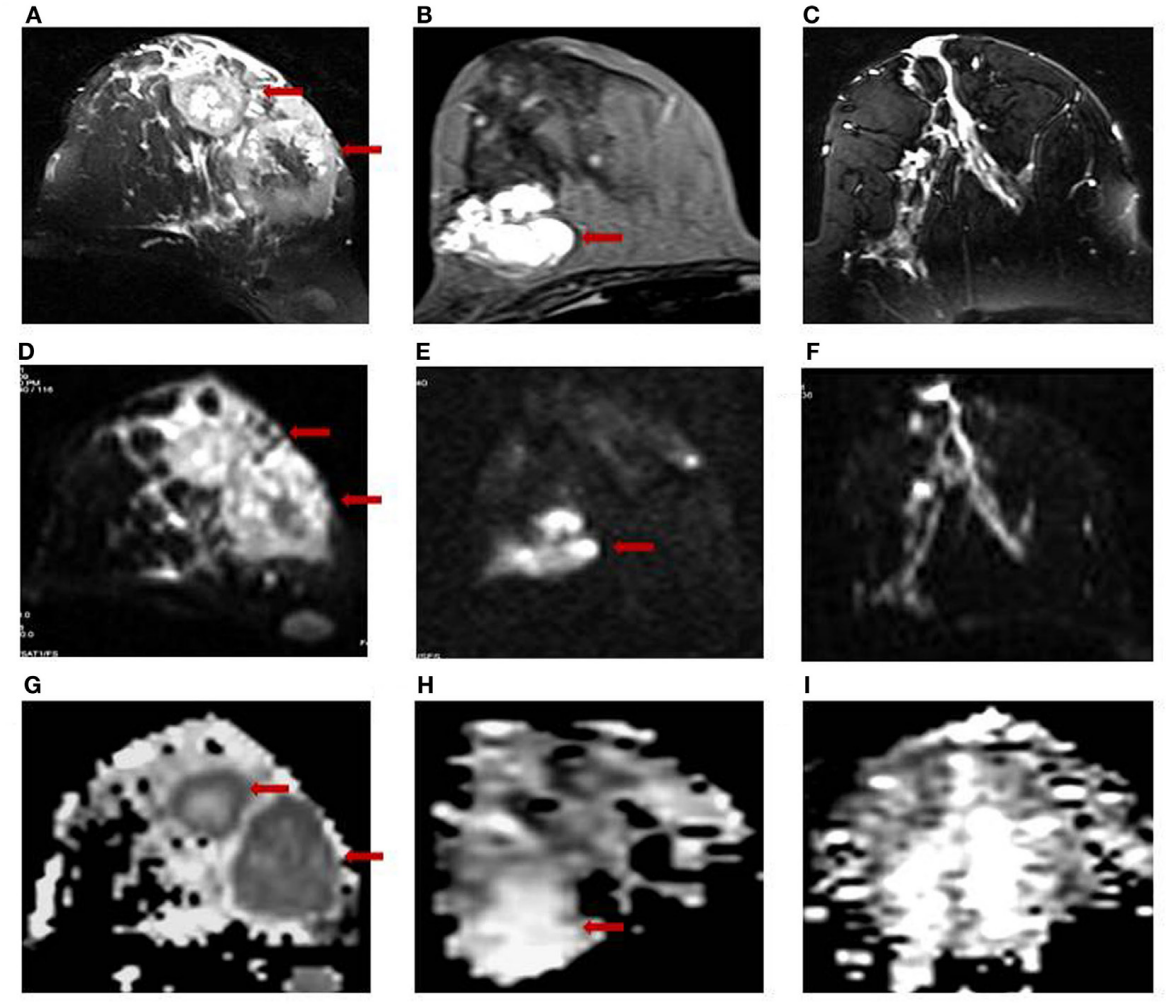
T2-weighted MR images of (A) a 28-year-old patient with infiltrating ductal carcinoma (IDC); (B) a 25-year-old patient with benign fibroadenoma; and (C) a 30-year-old volunteer with healthy breast tissue. The respective DW images are shown in (D–F), while the ADC maps obtained are shown in (G–I).
